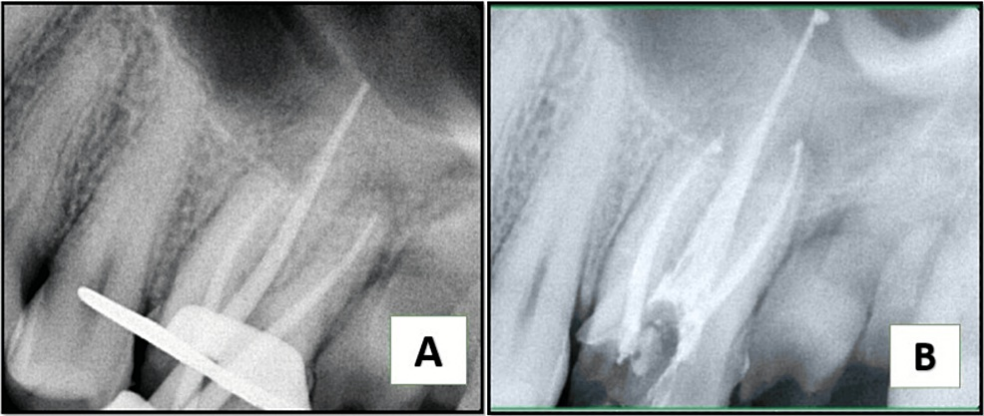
(A) Mastercone radiograph. (B) Immediate post-obturation radiograph.
